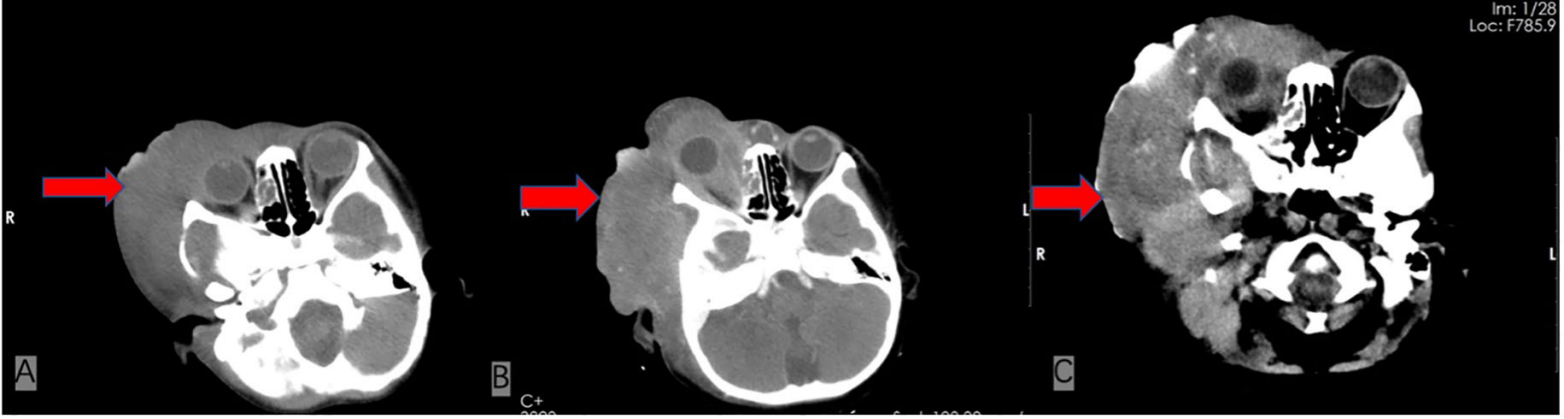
Comparison of serial cranial CT scans at the same axial level before and after treatment. (A) Contrast-enhanced CT from December 2018, at the time of admission, reveals a 4.6 cm × 11 cm × 11 cm mass in the right maxillofacial region with associated zygomatic arch bone destruction. (B) Follow-up CT in May 2019, after four cycles of chemotherapy, shows a newly developed metastatic lesion (4.9 cm × 12 cm) and narrowing of the external auditory canal. (C) Final CT scan from June 2019, shortly before the patient’s death, demonstrates partial tumor regression with increased calcifications.
